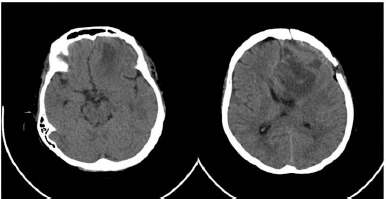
Complete removal of the tumour from serial brain CT
